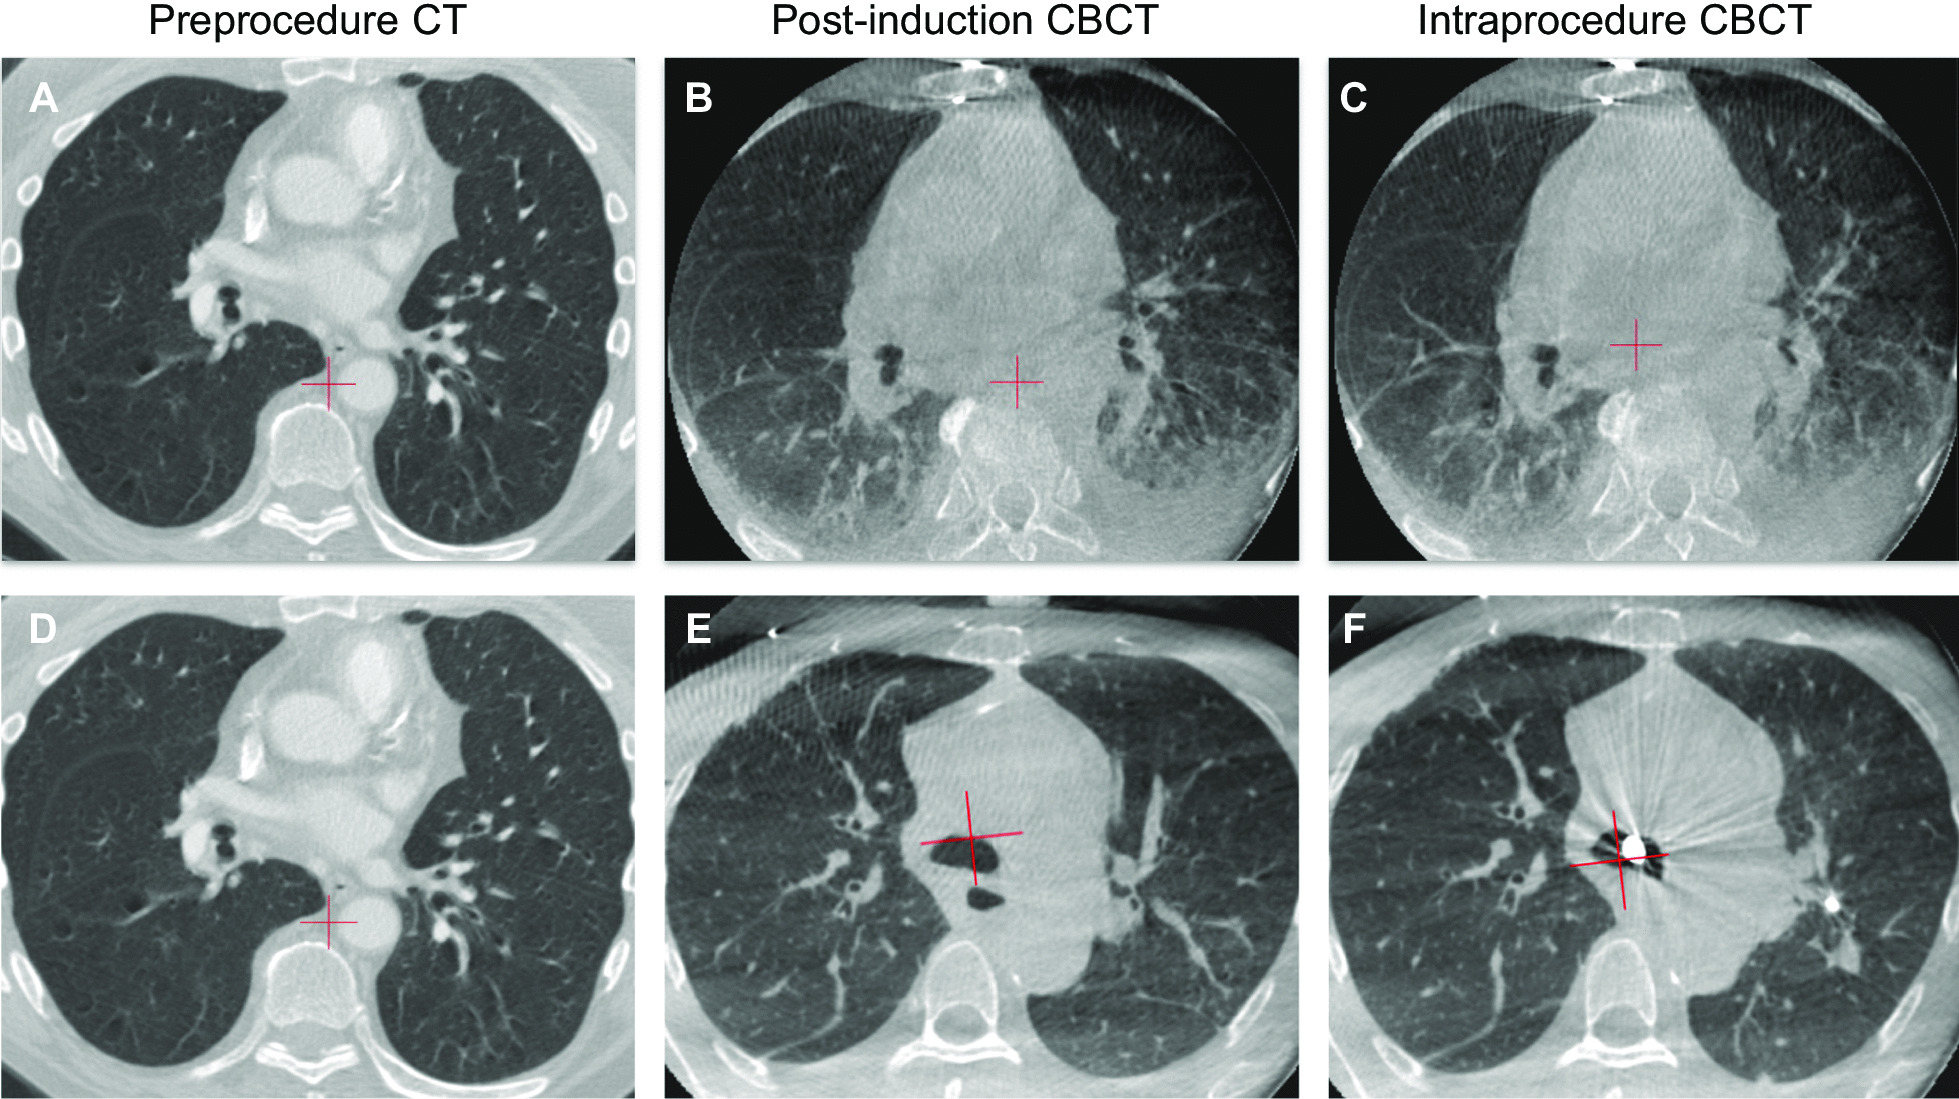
A–C Computed tomography (CT) and cone-beam CT (CBCT) scans during image-guided bronchoscopy without an optimized ventilation protocol. Significant atelectasis and ghosting artifact were observed. D–F CT and CBCT scans from a different patient using a ventilation protocol designed to prevent atelectasis
